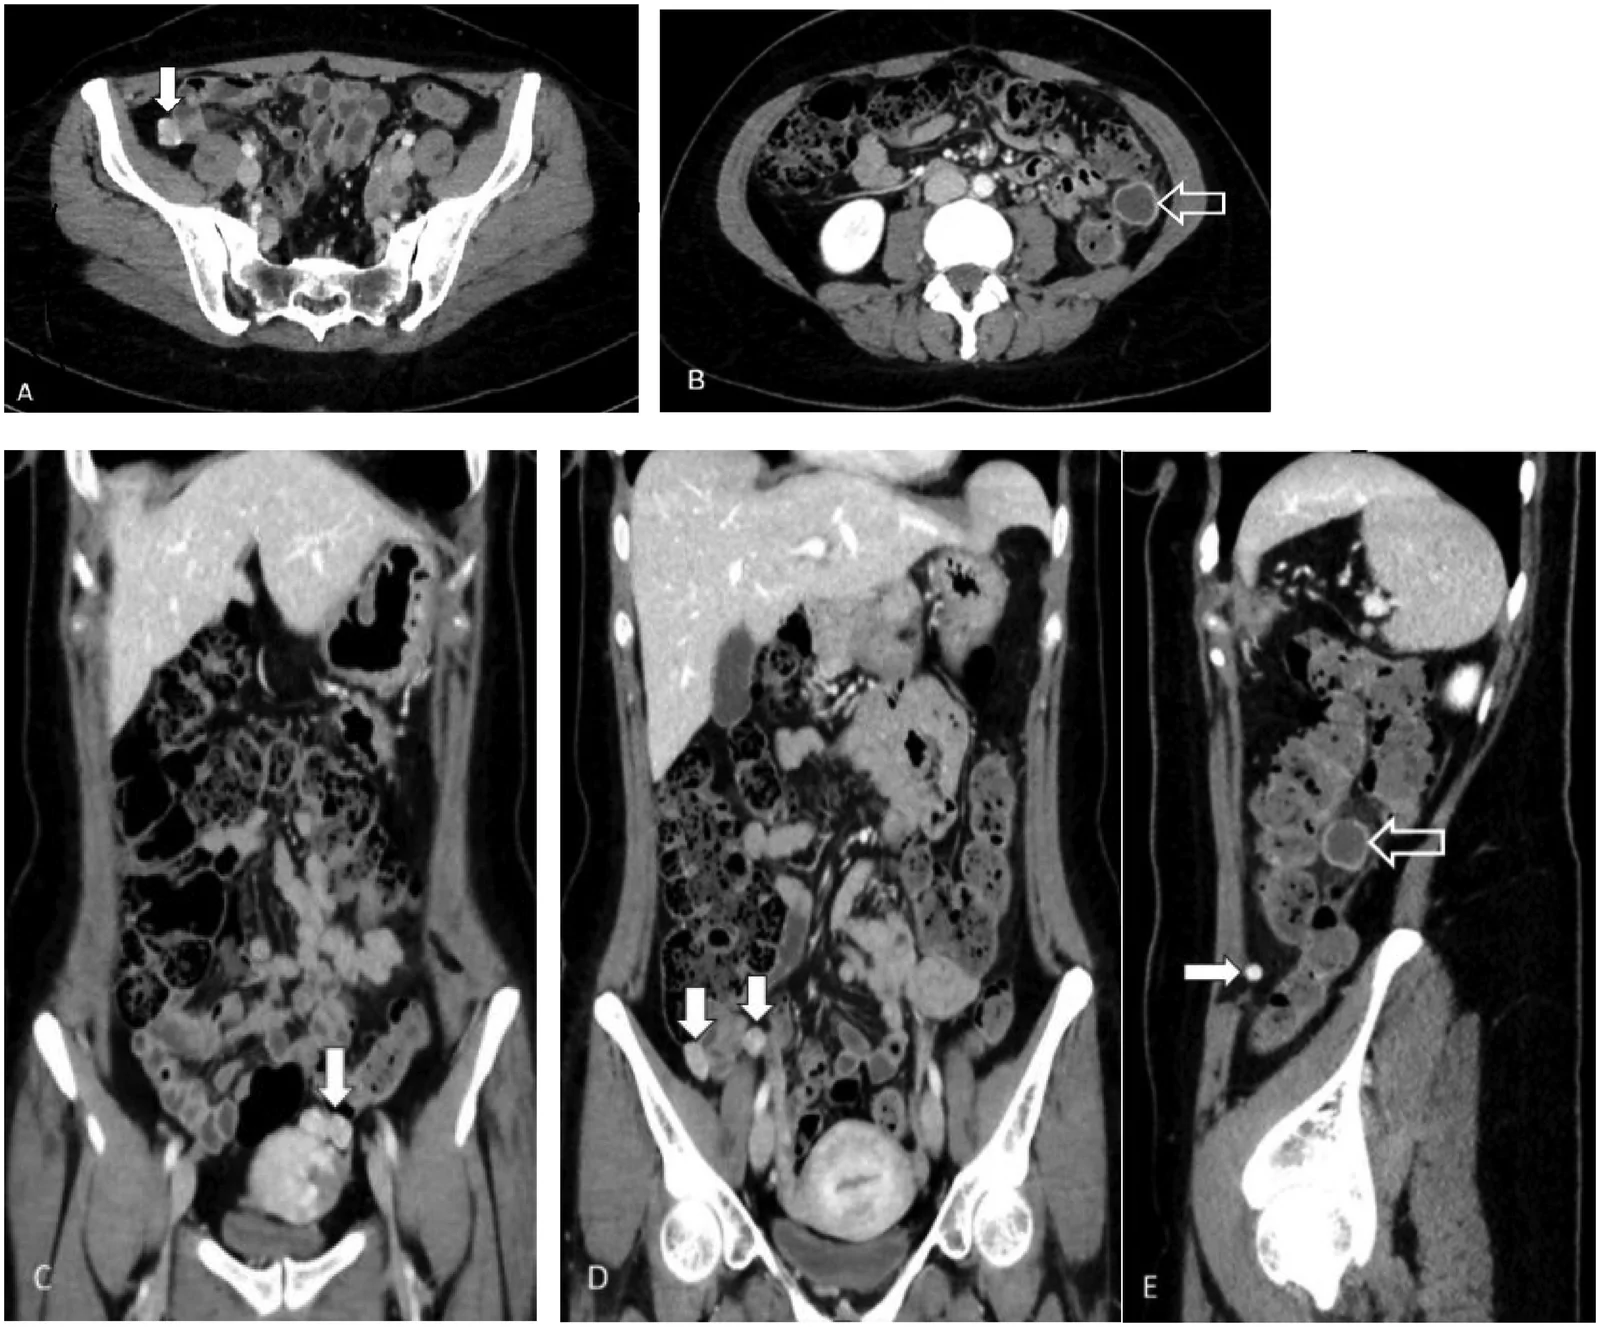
Enhanced CT of the abdomen and pelvis at the portovenous phase: (A) Axial: A solid peritoneal nodule is seen in the right iliac fossa adjacent to distal ileal loops. (B) Axial: A cystic nodule demonstrating fluid attenuation in HU values, with a thin enhancing rim is located at the left lateral aspect of the peritoneal cavity, insinuated between the mid-segment of the descending colon and the distal segment of the transverse colon. (C) Coronal: A solid homogeneously enhancing nodule is seen at the left superolateral aspect of the uterine operative bed. (D) Coronal: Two homogeneously enhancing solid nodules are located medial and lateral to distal ileal loops. (E) Sagittal: A cystic nodule (empty arrow) and a tiny solid peritoneal nodule (filled arrow).
